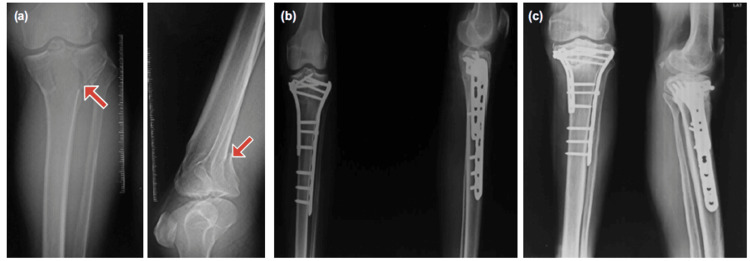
Radiographic evaluation of Schatzker type V tibial plateau fracture treated with dual plating (A) Preoperative AP and lateral radiographs showing a bicondylar tibial plateau fracture (Schatzker type V) involving both medial and lateral condyles (red arrows). (B) Immediate postoperative radiographs demonstrating anatomical reduction and internal fixation using dual locking plates via a two-incision approach. (C) Six-month follow-up radiographs revealed maintained reduction, implant position, and progressive fracture healing. AP: anteroposterior
